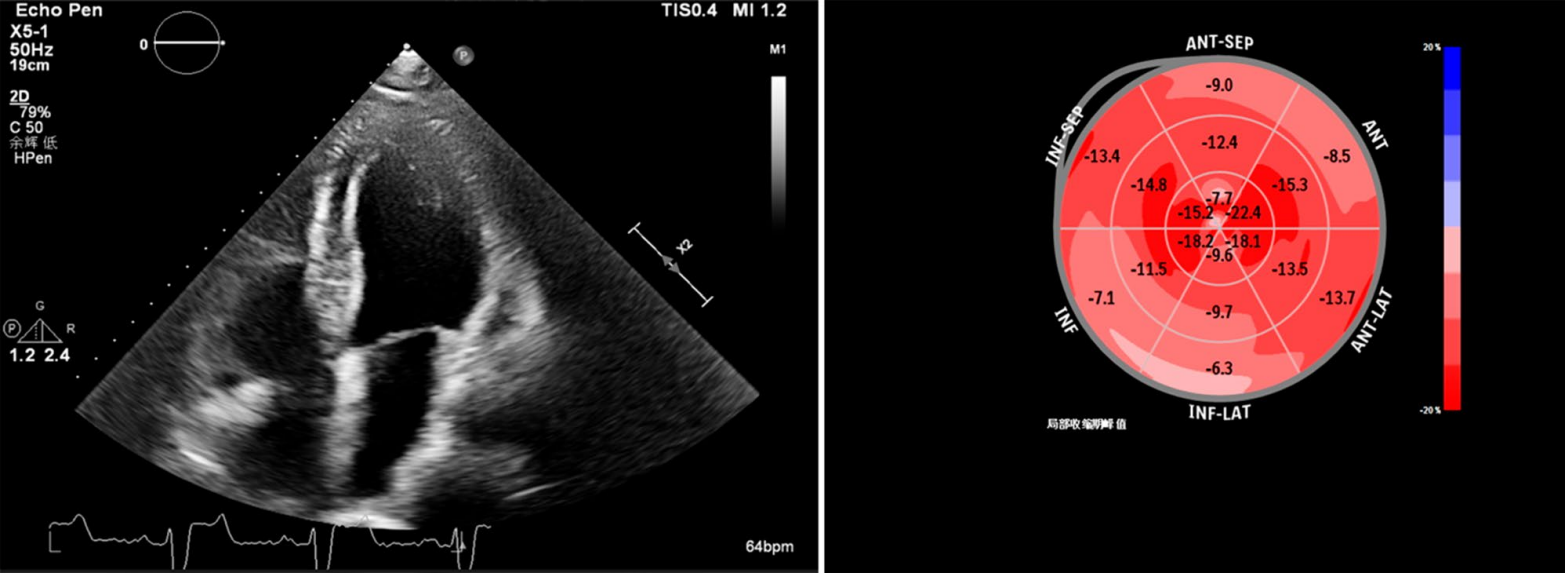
The three-dimensional echocardiography revealed a distinct bilateral sign in the ventricular septum, diffuse hypertrophy of the left ventricular wall, left ventricular dysfunction
